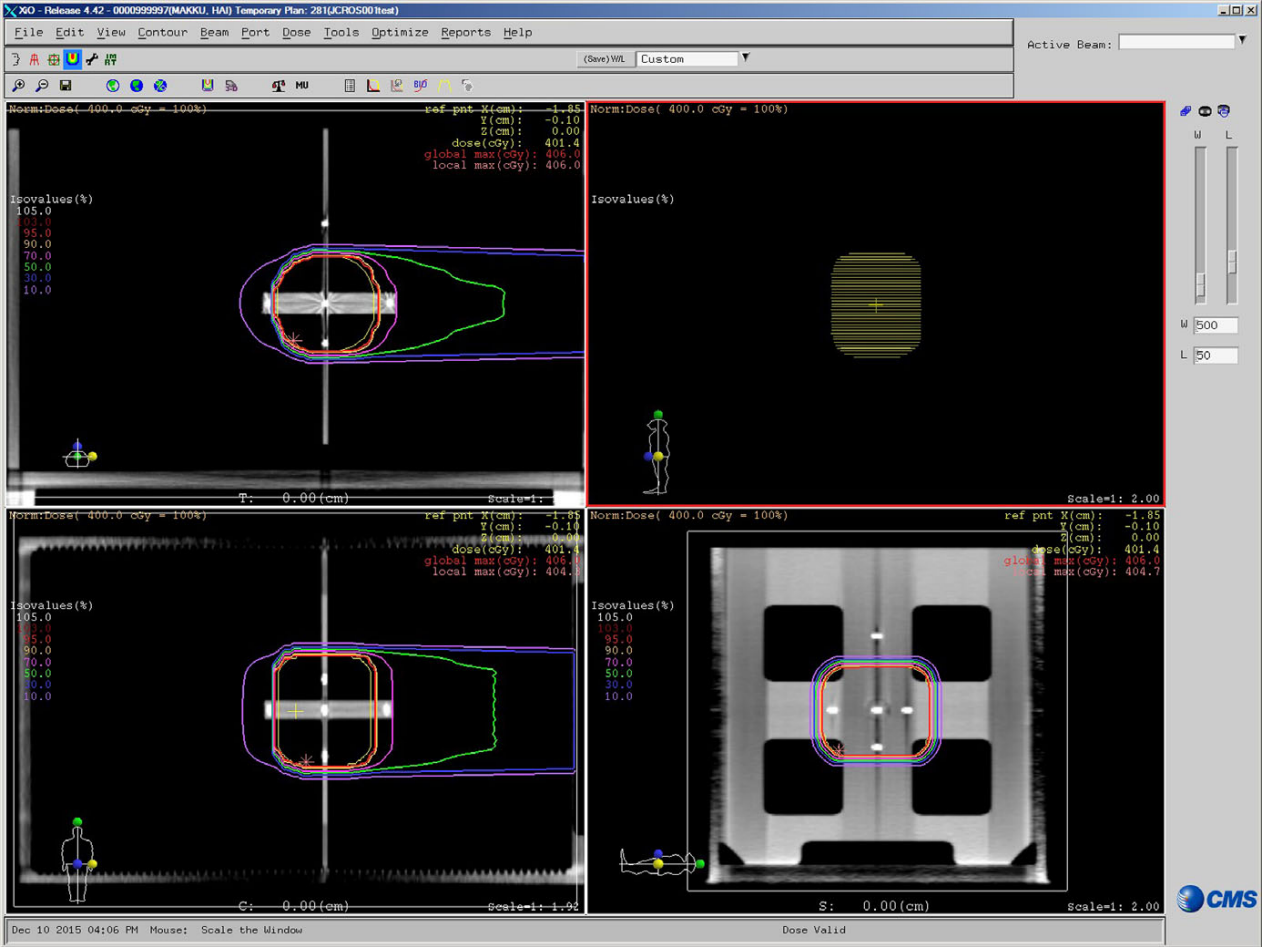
Screen shot of the treatment plan that was generated as per the audit criteria (Scanning beam). Left‐ upper window: Axial CT image, Left‐lower window: Coronal CT image, Right‐upper window: Beam's eye view, Right‐lower window: Sagittal CT image. The yellow contour line shows the PTV shape and CT images with the dose distribution.
